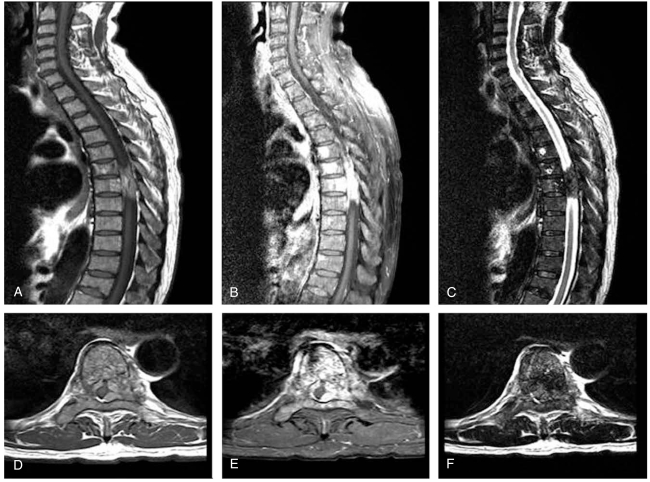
T1-weighted magnetic resonance imaging demonstrating a T7-8 epidural mass ventral to the spinal cord (A: sagittal, D: axial) with high enhancement (B: sagittal, E: axial); the lesion showed low signal intensity on the T2-weighted images (C: sagittal, F: axial).
